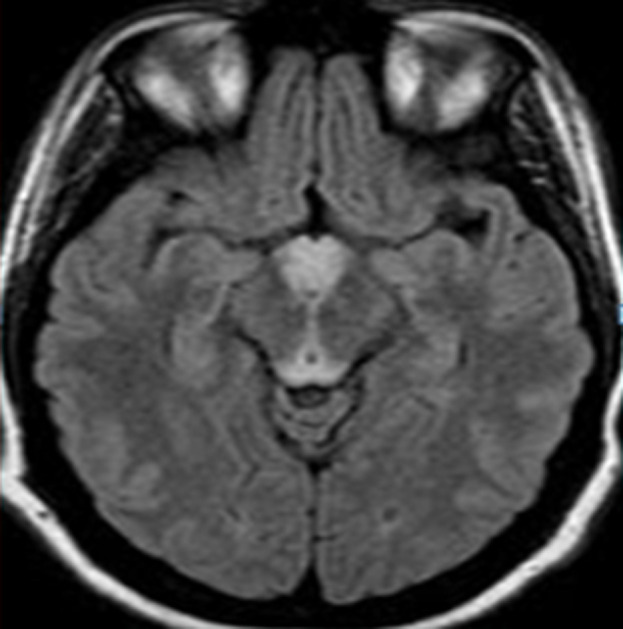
IRM encéphalique, en coupes axiale séquence FLAIR, objectivant des hyper signaux au niveau des corps mamillaires, en péri-acqueducal
